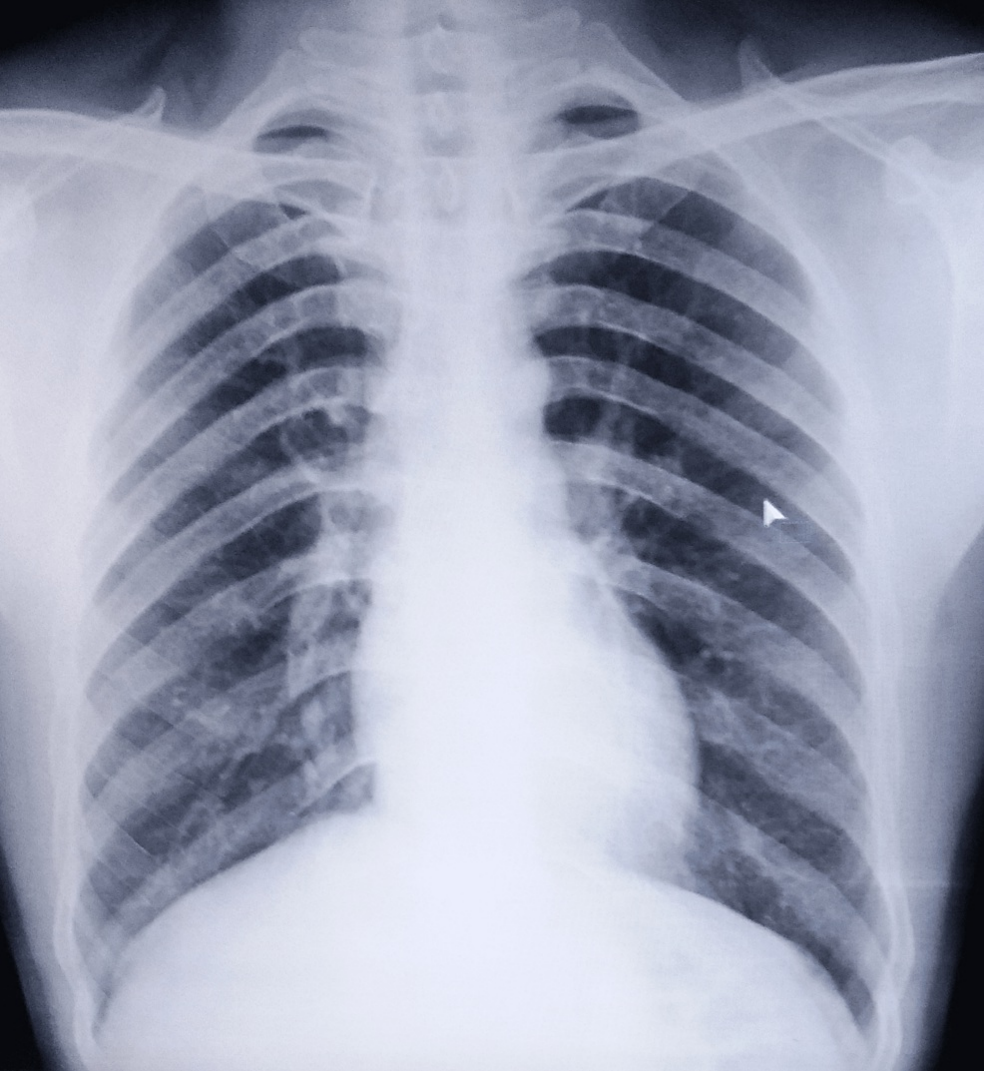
A chest radiograph not suggestive of pulmonary tuberculosis
